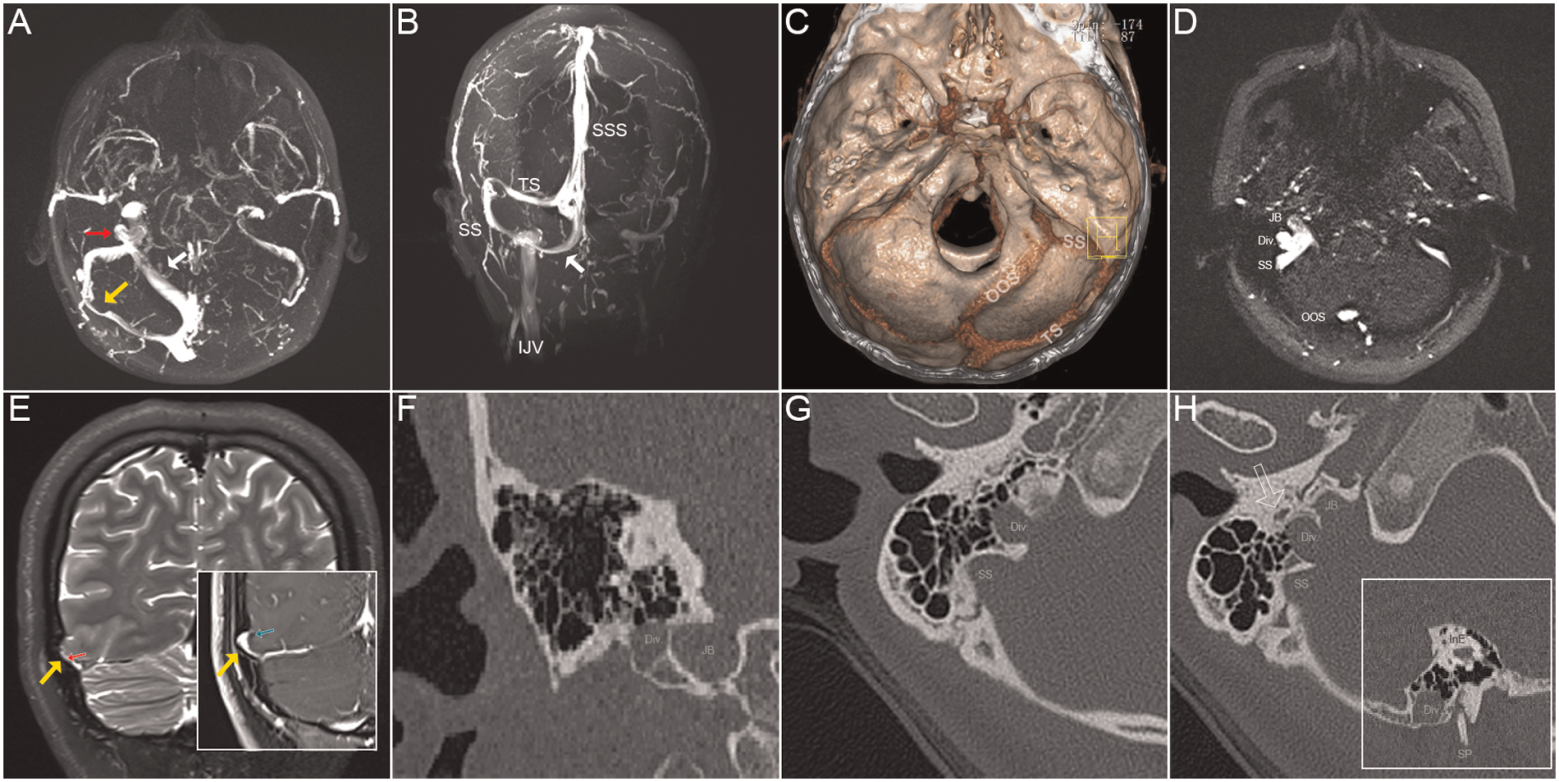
Radiological imaging and signs of case 1 of right-sided pulsatile tinnitus. (A) The maximum intensity projection (MIP) reconstruction of axial 2D-time-of-flight (TOF) magnetic resonance (MR) images showing an enlarged oblique occipital sinus (OOS, white arrow) anastomosing with the lower curve sigmoid sinus (SS), forming a diverticulum (red arrow) in between the SS and jugular bulb (JB). Note the abrupt transverse sinus stenosis at the middle segment. (B) Coronal MIP reconstruction of 2D-TOF MR images. The white arrow indicates the enlarged OOS. (C) Volume rendering technique reconstructed using postoperative contrast-enhanced CT images demonstrating an enlarged OOS running above the occipital bone and underneath the cerebellum to directly anastomose with SS. (D) An axial 2D-TOF MR slice showing the SS, diverticulum (Div.), JB, and OOS. (E) Coronal T2-weighted MR and T1-weighted 2D FLASH images showing the brain herniation into arachnoid granulation [(cerebrospinal fluid signal hyperintense on T2 (red arrow) and hypointense on T1 (green arrow)] that compress into the transverse sinus lumen (yellow arrow), which should be suspected of increased intracranial pressure. (F) Coronal CT image showing the relative location of the diverticulum and JB. (G) Axial CT image exhibiting a seemingly right-sided high-riding JB dehiscence/diverticulum (pseudo-JB anomalies sign) invading the mastoid cavity. The bulging vascular wall emerging at the lower SS was a diverticulum (Div.) but not JB. (H) Axial CT slice showing the SS, diverticulum (Div.), and JB. The hollow arrow indicates the facial nerve canal. The reconstructed sagittal CT slice shows the diverticulum (Div.) nearly exited the mastoid apex. SP and InE indicate the styloid process and inner ear, respectively.
